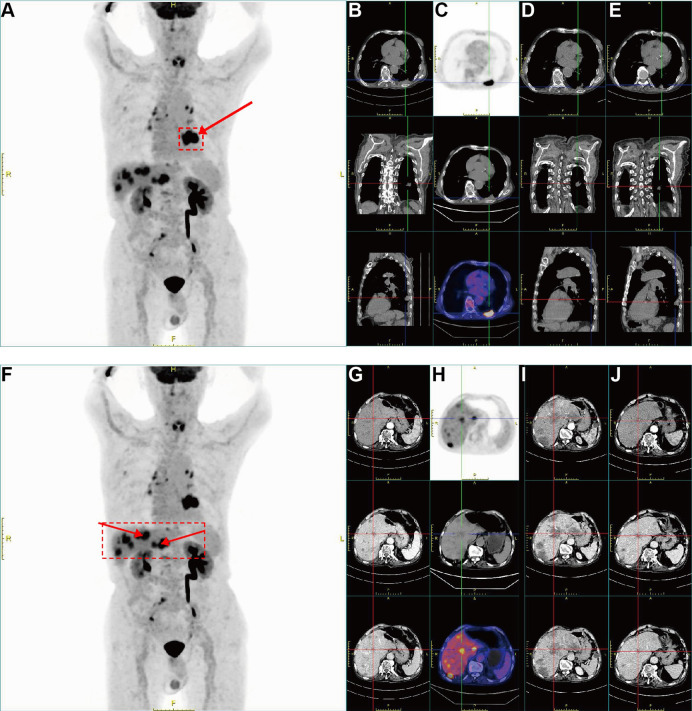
Baseline 18F-FDG PET/CT and serial CT follow-up demonstrating discordant response between the primary lung lesion and hepatic metastases. (A) Whole-body maximum-intensity-projection (MIP) 18F-FDG PET image at baseline; arrow indicates the hypermetabolic primary lesion in the left lower lobe (dashed box highlights the lesion-bearing region). (B) Axial chest CT at baseline showing a patchy soft-tissue–attenuation lesion in the peripheral left lower lobe with marked emphysema and mildly enlarged mediastinal lymph nodes. (C) Corresponding axial PET image confirming prominent FDG uptake in the pulmonary lesion. (D) Follow-up chest CT after NSCLC-directed therapy (pembrolizumab plus vinorelbine) showing minimal change, consistent with stable disease, in the pulmonary lesion. (E) Follow-up chest CT after switching to etoposide plus carboplatin showing stable-to-slightly decreased pulmonary lesion. (F) Whole-body MIP 18F-FDG PET image at baseline highlighting FDG-avid hepatic metastases; arrowheads indicate representative hypermetabolic liver lesions (dashed box outlines the liver-dominant disease region). (G) Baseline abdominal CT demonstrating multiple hypodense lesions in both hepatic lobes. (H) Corresponding axial PET and fused PET/CT images showing marked FDG hypermetabolism of the hepatic lesions. (I) Follow-up abdominal CT after pembrolizumab plus vinorelbine showing rapid progression of liver metastases (increased size/number). (J) Follow-up abdominal CT after etoposide plus carboplatin showing marked regression of multiple hepatic metastases, with some lesions nearly resolved.
